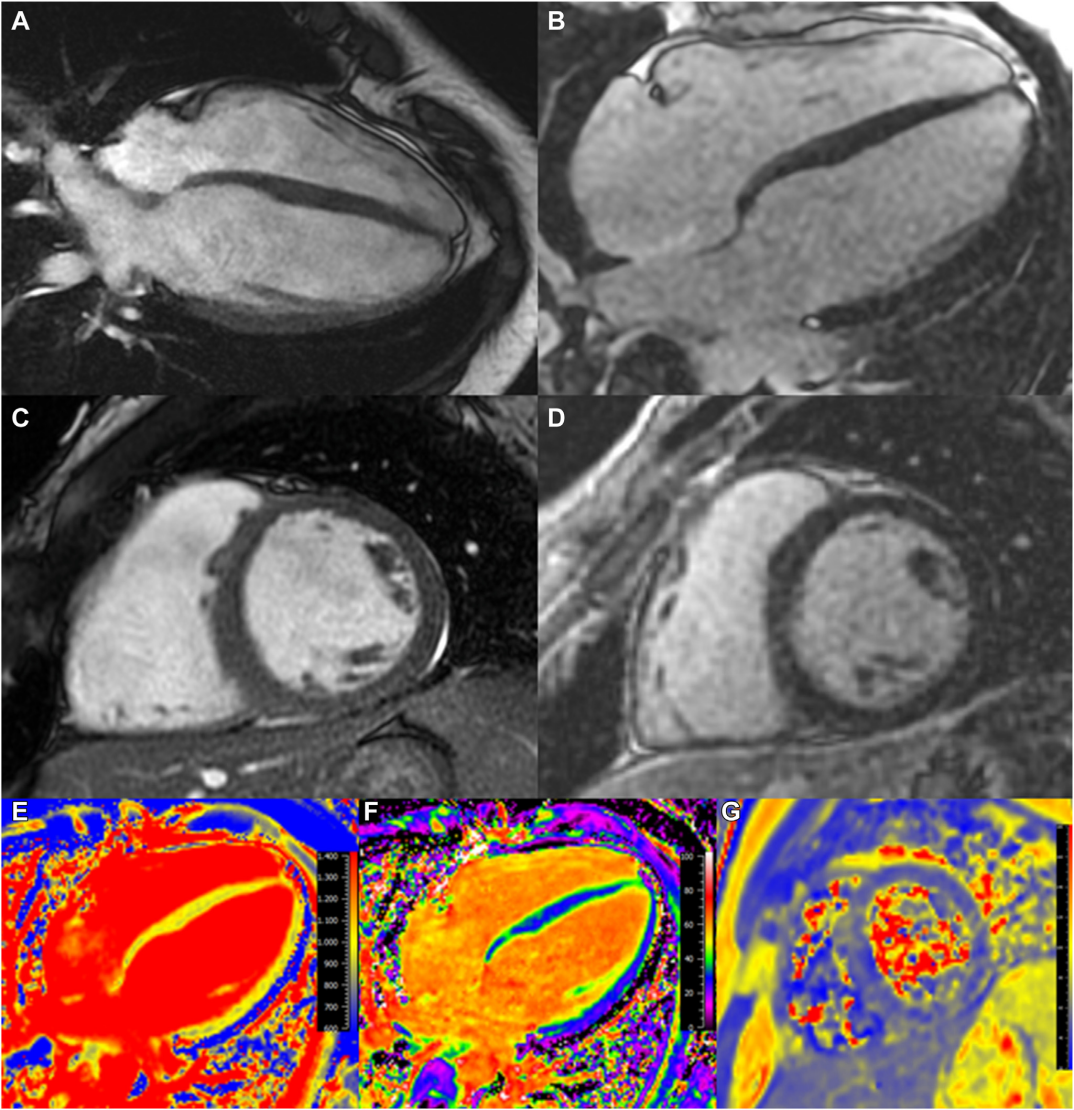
Cardiovascular magnetic resonance imaging. End-diastolic steady-state free precession cine images from the (A) 4-chamber and (C) mid-ventricular short-axis views. B, D: Corresponding late gadolinium enhancement (LGE) images. Note the lack of LGE. E, F: T1 mapping with normal T1 and extracellular volume (ECV) values (basal septal native T1 value of 978 milliseconds with an ECV of 30.9%, both within normal limits for our scanner (Signa Artist 1.5T, GE Healthcare).^8^G: T2 mapping of the short-axis myocardium showing normal T2 values (mid-myocardial 50 milliseconds).
